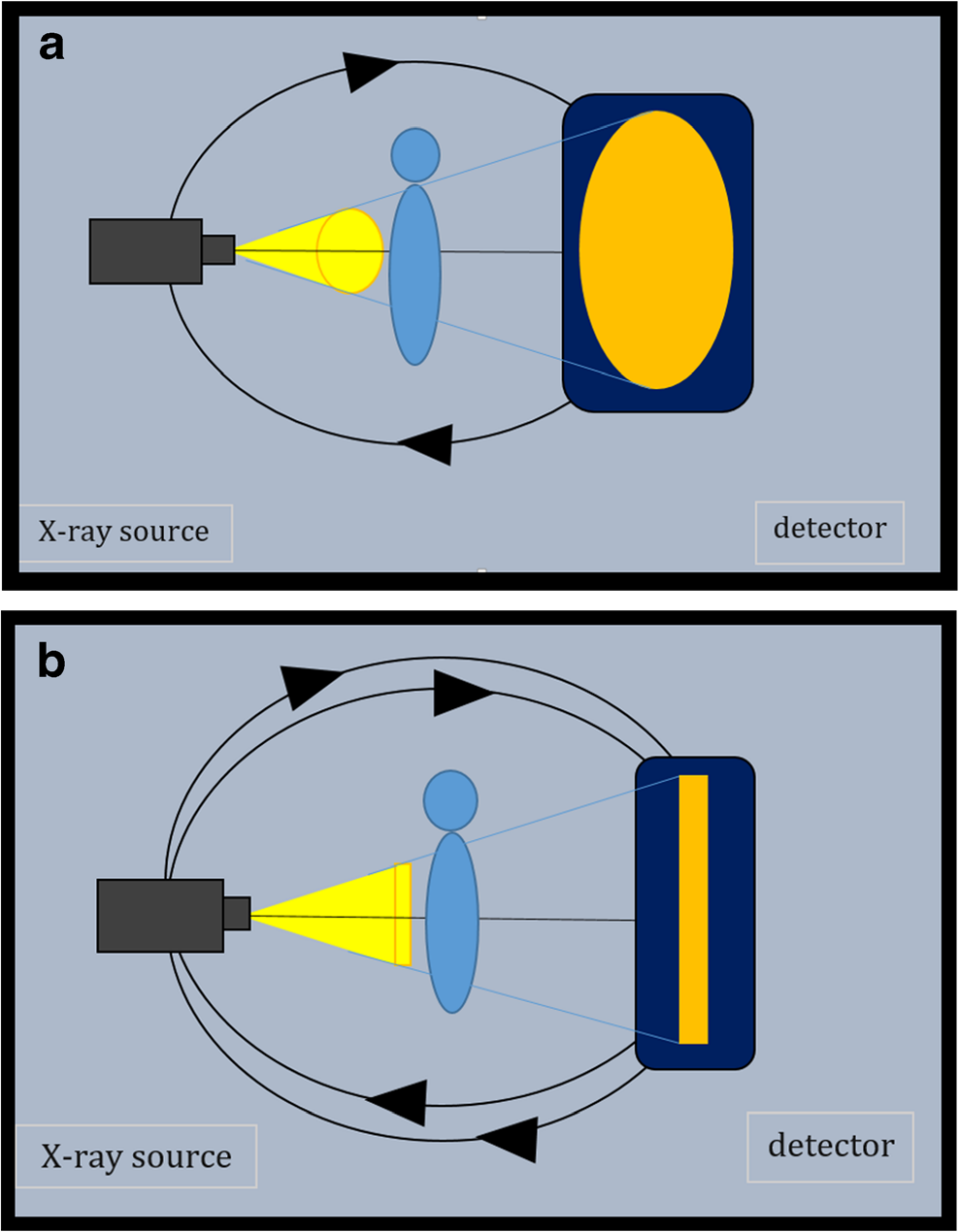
Principle of CBCT and MDCT. a In CBCT, cone-shaped X-ray beam reaches a flat detector after a single rotation of the gantry around the patient. b In MDCT, narrowly collimated, fan-shaped beam and multiple linear detectors rotate around the patient to acquire multiple image sections per rotation. In both techniques volumetric images are reconstructed into a 3-D volume dataset of images
